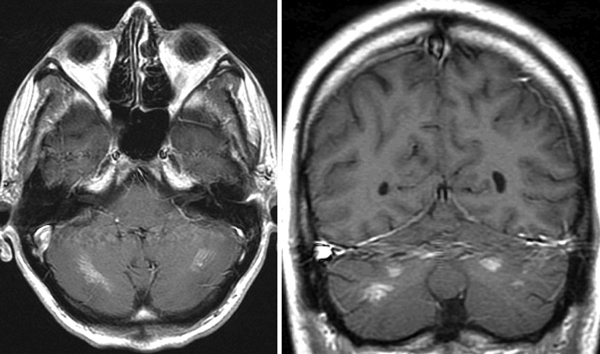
MRI scan (axial and coronar) with symmetric contrast agent enhancement in the cerebellum (white spots).
